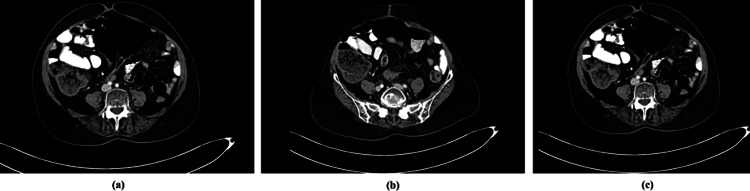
Contrast CT scan showed heterogeneous bilateral adnexal masses consistent with malignant neoplasm, omental and peritoneal metastatic deposits, ascites and enlarged retroperitoneal and pelvic nodes (brown arrow).
